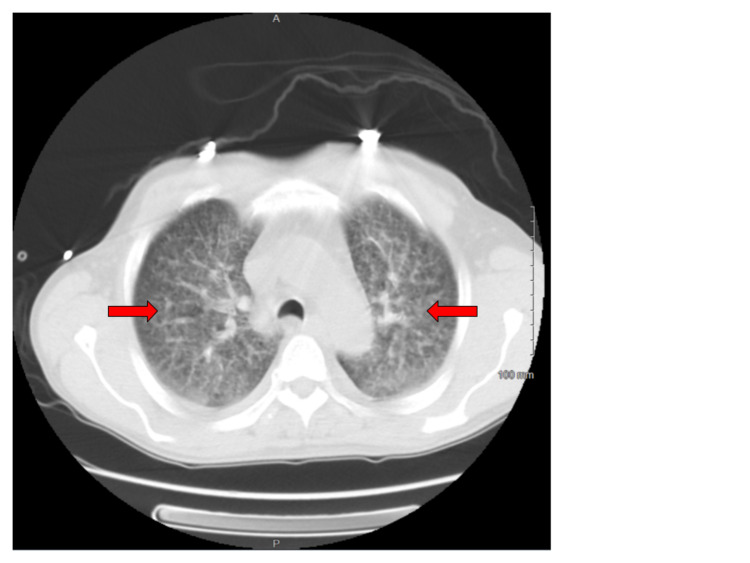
CT scan of the chest (axial view, lung window) at the level of trachea showing the extensive distribution of bilateral reticulonodular and bilateral ground glass opacities (red arrow) consistent with acute histoplasmosis in the patient with HIV.
